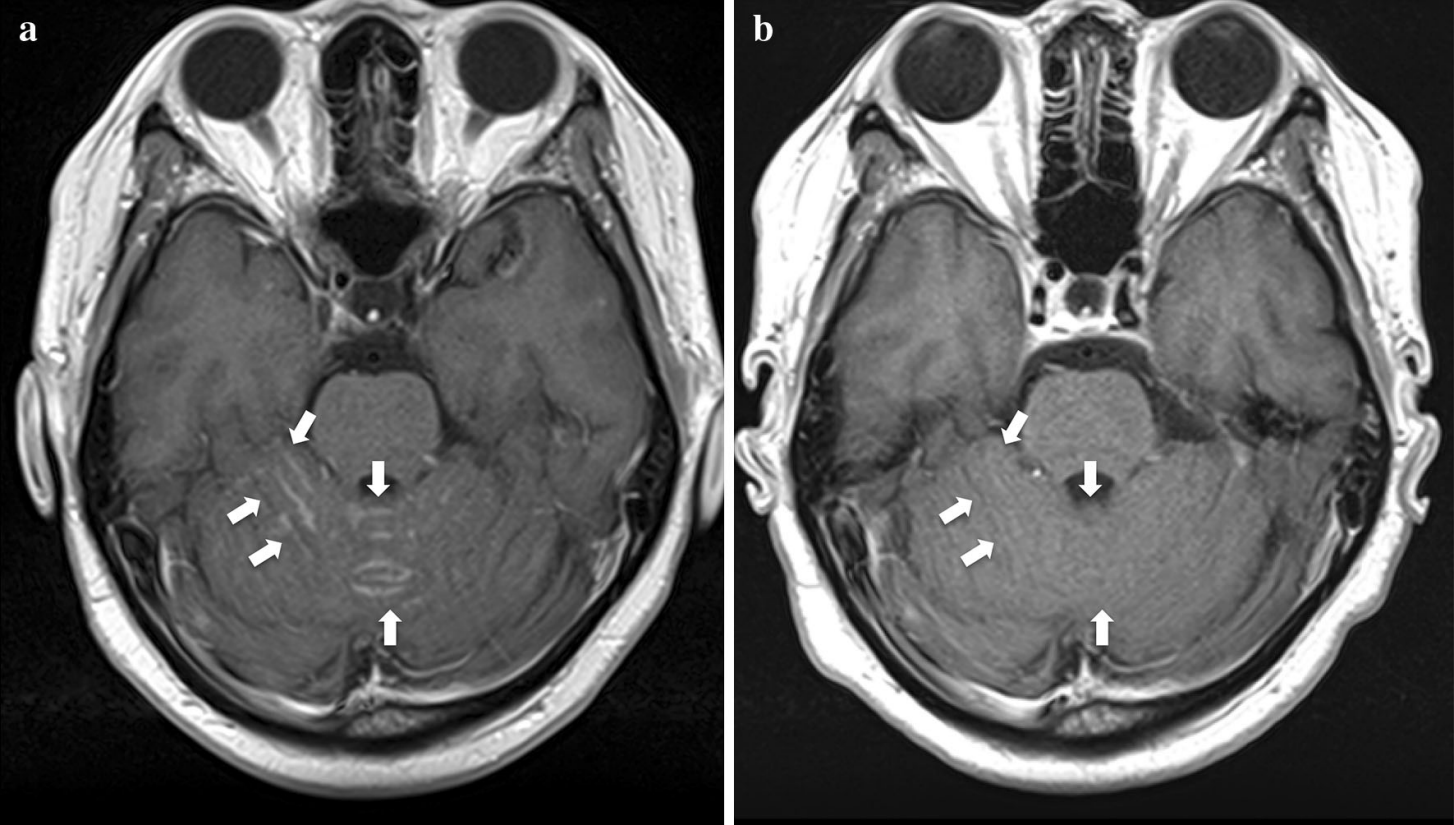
T1-weighted post-gadolinium MRI before a and after b 3 months of alectinib treatment revealed a marked response in leptomeningeal carcinomatosis (see *arrows*)
